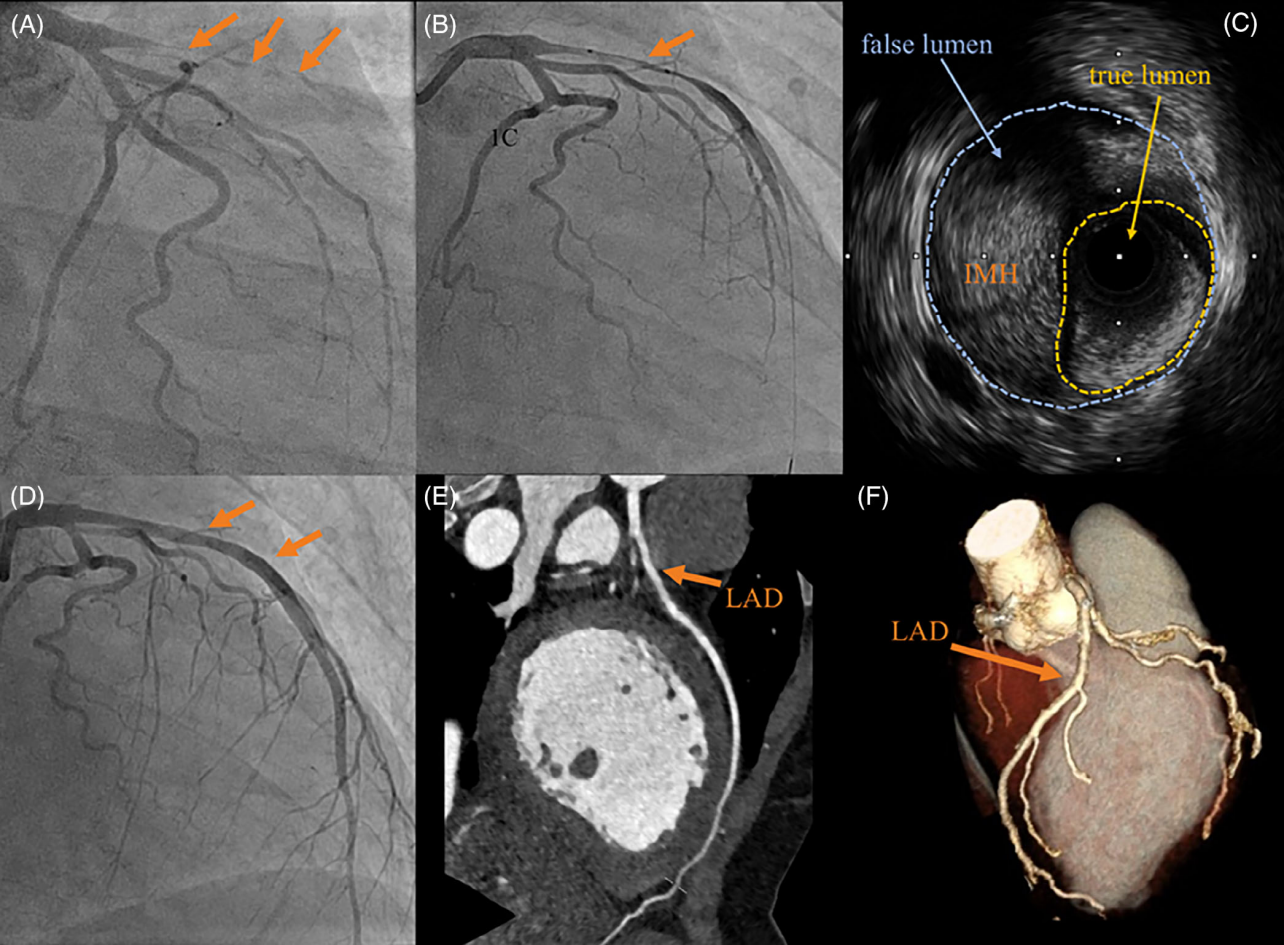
(A, B) Angiography showing left anterior descending artery (LAD) dissected with no flow on initial presentation, and then some flow return with gentle balloon angioplasty. (C) Intravascular ultrasound of the same LAD revealed the intramural hematoma (IMH) compressing the true lumen. (D) Angiogram showing good final results after angioplasty, no stent implanted. (E, F) Follow‐up computed tomography showed 0 calcium score and no significant stenosis of the LAD (arrows depict site of previous dissection)
